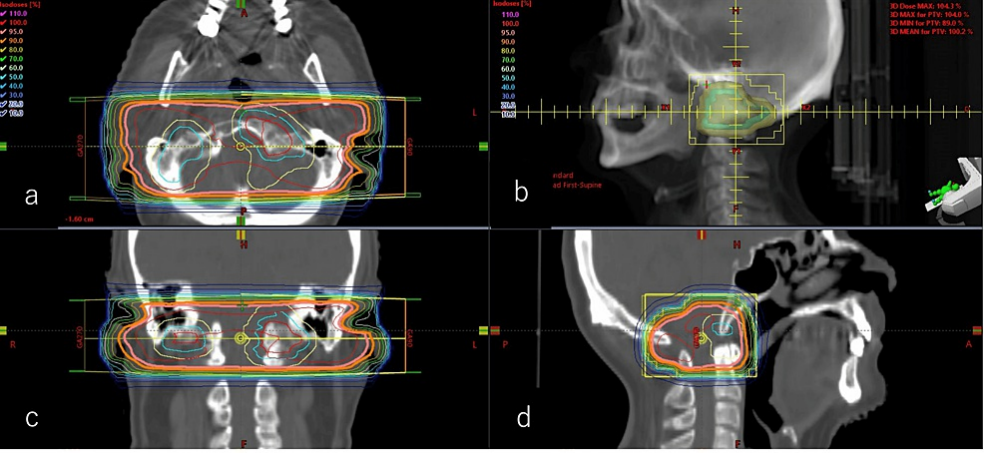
Palliative radiation therapy to alleviate symptoms. Treatment planning was performed using Eclipse (Palo Alto, CA: Varian Medical Systems). Radiotherapy fields are shown in (a) axial image, (b) beam's eye view, (c) coronal image, (d) and sagittal image. The gross tumor volume is outlined by red lines for tumors on both the left and right sides of the occipital bone metastases. The clinical target volume and planning target volume are represented by light blue and yellow lines, respectively. X-ray beams from two directions were performed.
